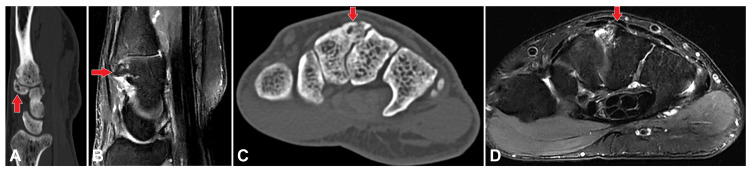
(A)-(D) A marker was placed at the point of interest, and both CT axial imaging and 3T MRI were performed. The provided images include axial and sagittal sections of the MRI (PD fat-saturated images) and the CT scan. These images demonstrate hypertrophied bony protuberances at the base of the third metacarpal and trapezoid bones, forming a pseudoarthrosis with osteoarthritic changes, marrow edema, and subcortical tiny pseudocystic areas. The findings of hypertrophied bony protuberances at the base of the third metacarpal and trapezoid bones, along with the associated pseudoarthrosis, osteoarthritic changes, marrow edema, and subcortical tiny pseudocystic areas, are noted. MRI: Magnetic resonance imaging; CT: Computed tomography; PD: Proton density
